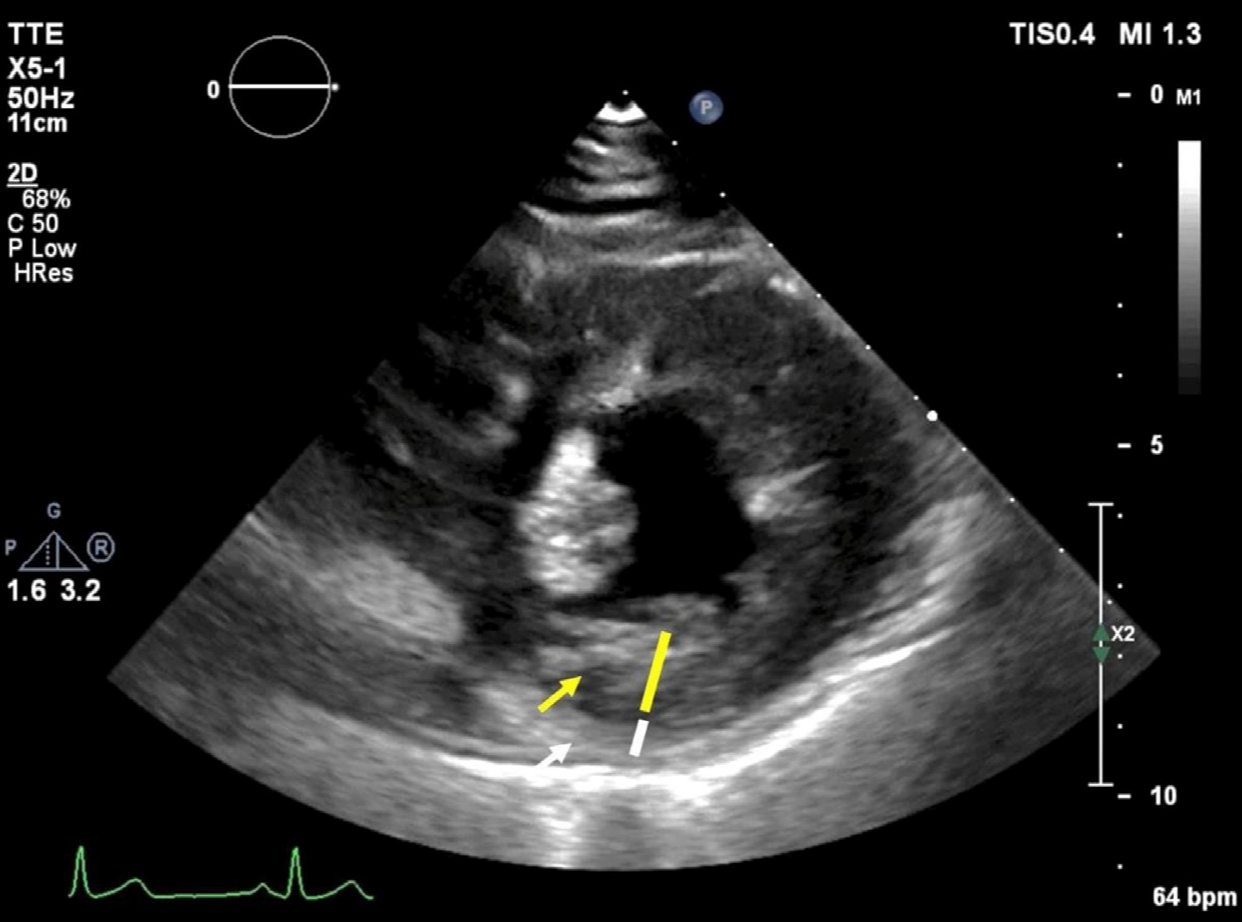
Transthoracic echocardiography demonstrates a ratio of the systolic thickness of the noncompacted (yellow line) to compacted layer (white line) greater than two in the parasternal short axis view.
